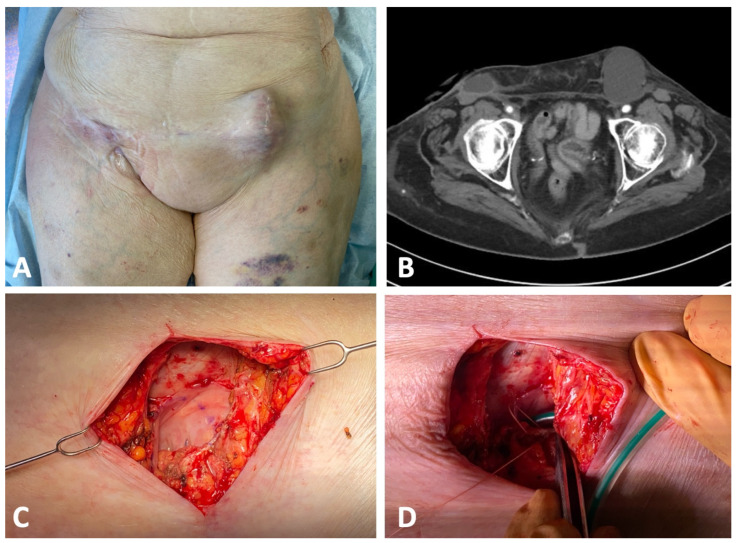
Patient suffering from one lymphocele in each groin. (A): Open lymphocele in the right groin; closed lymphocele in the left groin. (B): CT scan of the patient. (C): Reduced left lymphocele after partial resection. (D): Reduced lymphocele with inlying drain.
